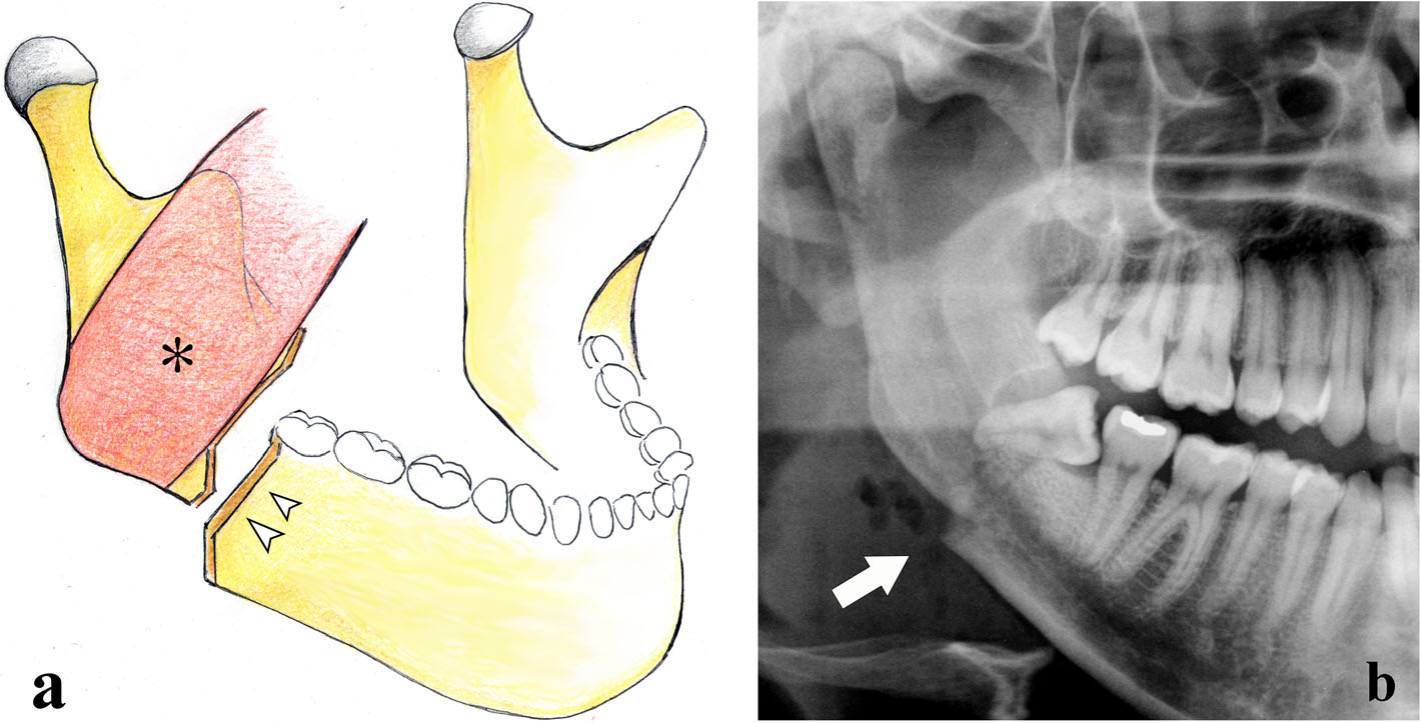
Angle fracture. a Picture showing a vertical fracture that runs distally to the third molar (arrowheads). It is a displaced fracture since the masseter muscle (asterisk) pulls the distal bone fragment upwards and medially. b Cropped panoramic radiograph. Mandibular angle fracture involving an impacted third molar (arrow)
